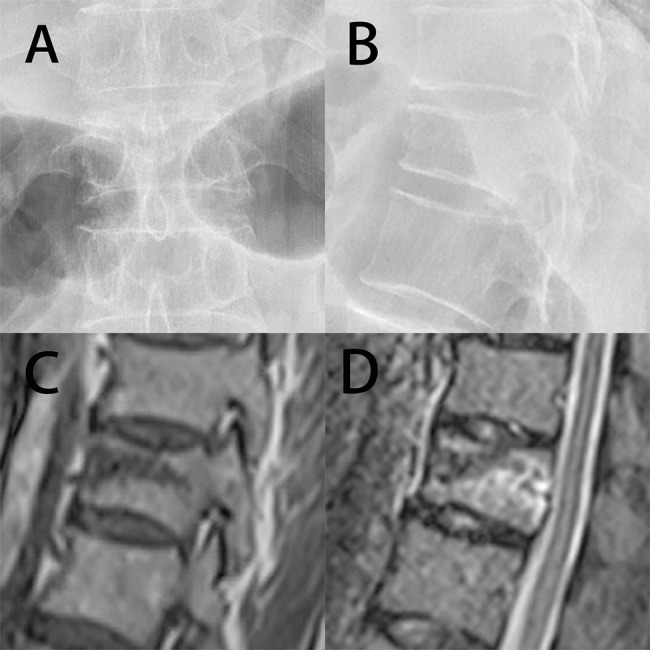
Typical X-ray and magnetic resonance (MR) images of OVCFs. A and B, Posterior-anterior and lateral X-ray films showing a compression fracture in T12. Both endplates were fractured. C and D, MR images showing marrow edema in the fractured vertebral body.
